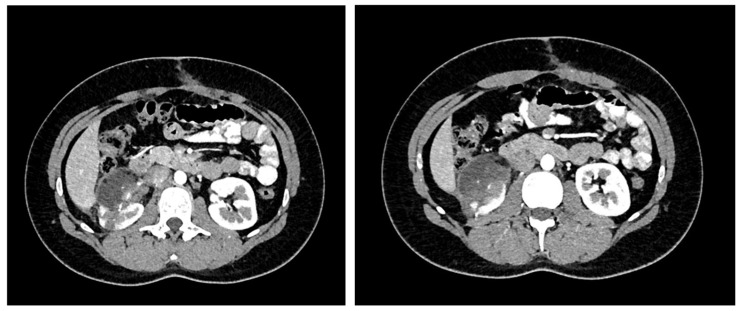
Preoperative computerized tomography (CT): Interpolarly localized isodense 6.8 cm × 6.2 cm tumor propagating to the renal sinus.
